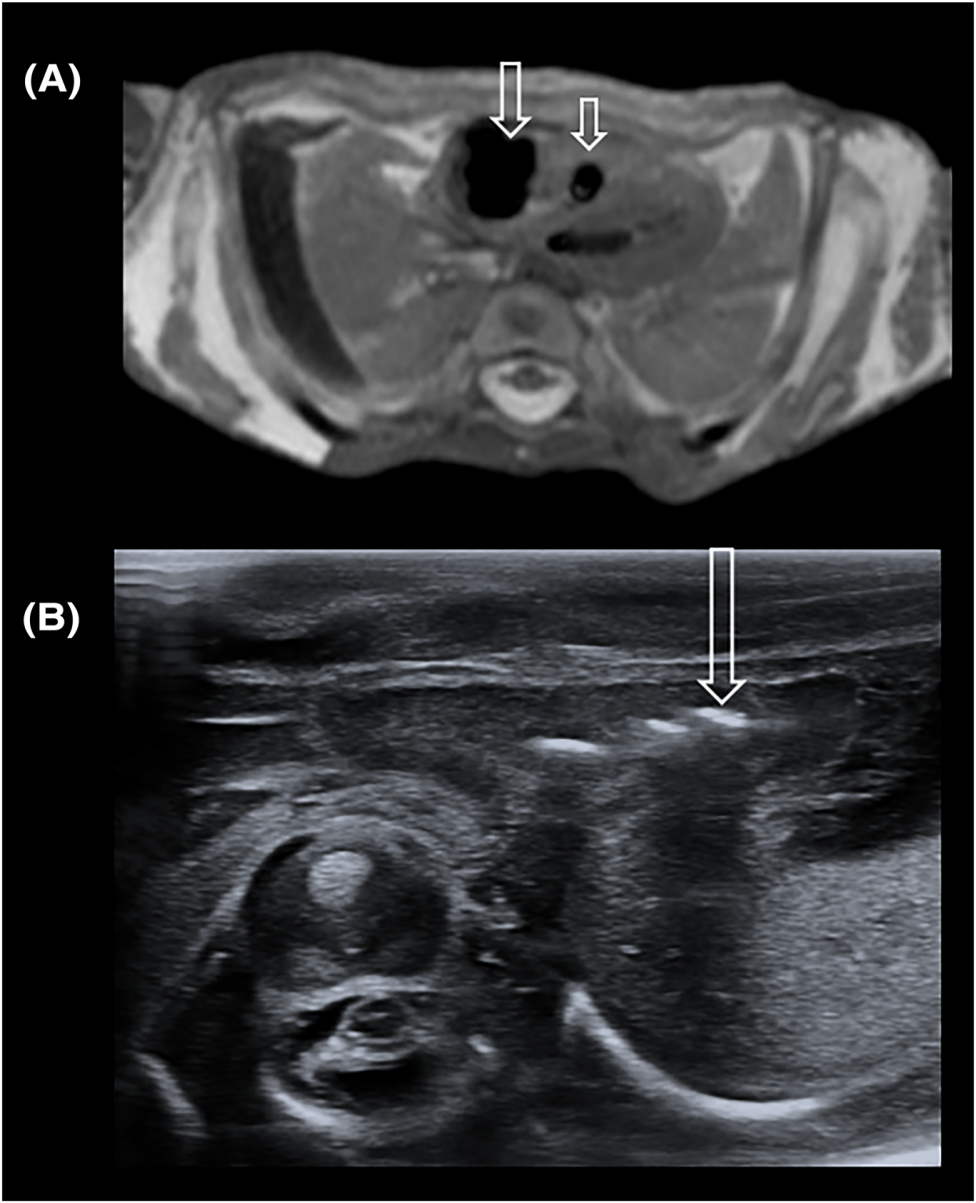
Nondiagnostic post‐mortem ultrasound cardiac images in a 25‐week gestational aged fetus. (A) Axial T2‐weighted post‐mortem MRI of the heart reveals multiple gas locules in the right atrium and ventricle (open arrows), which obscure sonographic wave penetrance and thus visualisation of the cardiac chambers on the (B) transverse ultrasound image. On the ultrasound image, intracardiac locules of gas are identified as white linear echogenic artefacts with posterior acoustic shadowing (open arrow) [Colour figure can be viewed at http://wileyonlinelibrary.com]
